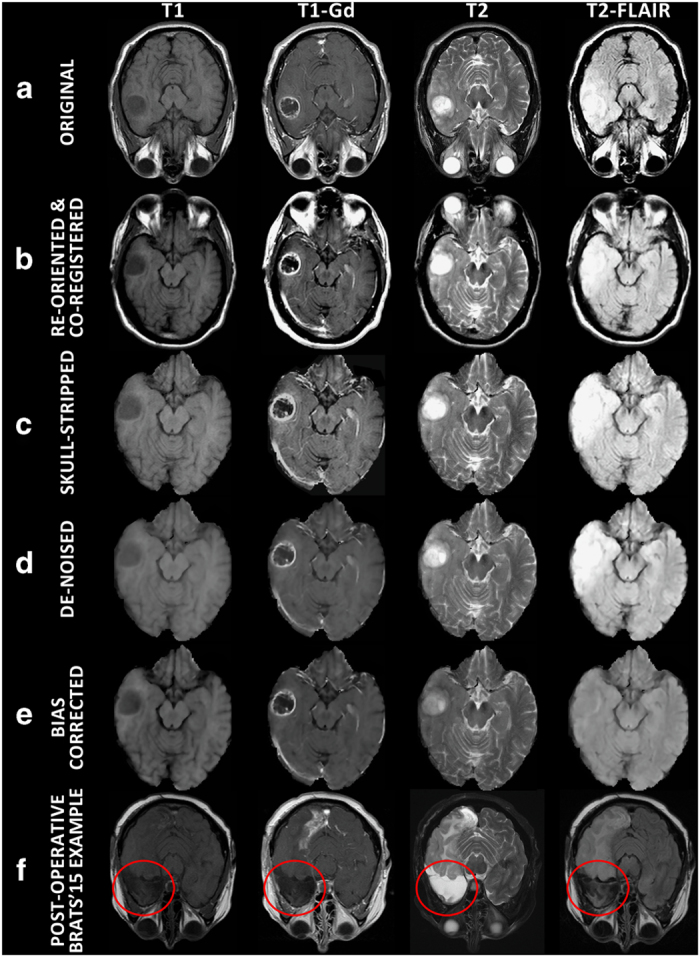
Single slice multimodal (T1, T1-Gd, T2, T2-FLAIR) MRI scans of example subjects. Examples are shown (a) in the original TCIA volume; (b–e) after application of various pre-processing steps; (f) for post-operative volumes in the BraTS ’15 data. Note that the step shown in (e), which is usually used to correct for intensity non-uniformities caused by the inhomogeneity of the scanner’s magnetic field during image acquisition, was not applied in the current data as it obliterated the T2-FLAIR signal.
